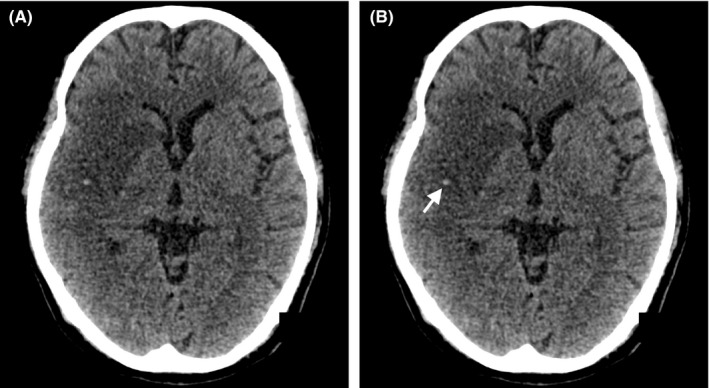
A, Nonenhanced cranial CT showing right‐sided MCA territory infarct. B, A hyperdense punctate focus (arrow) is noted in the right Sylvian fissure, corresponding to the MCA dot sign
